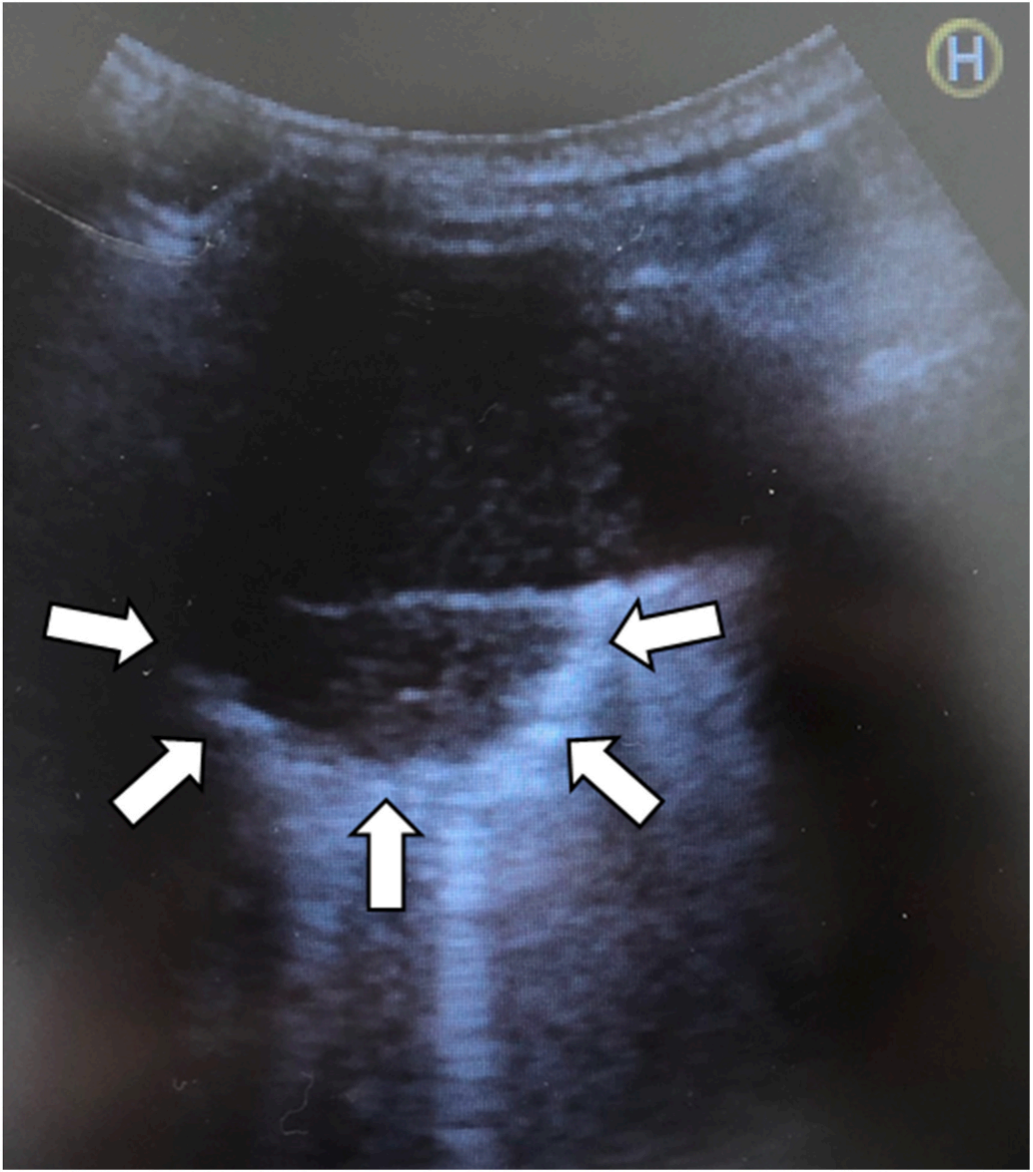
Transthoracic ultrasonography revealed a lesion in contact with the pleura of the left lung (white arrows).
